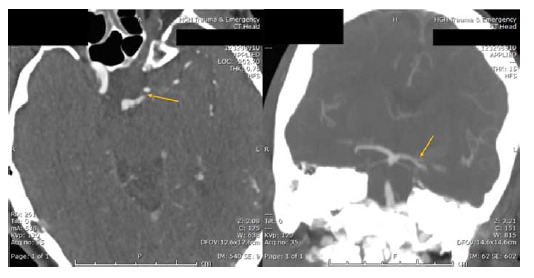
Computed tomography angiography of the head. There is a filling defect noted at the origin of the Percheron artery from the left P1 segment. This defect is clearly visible in both coronal and axial cuts (yellow arrows).
